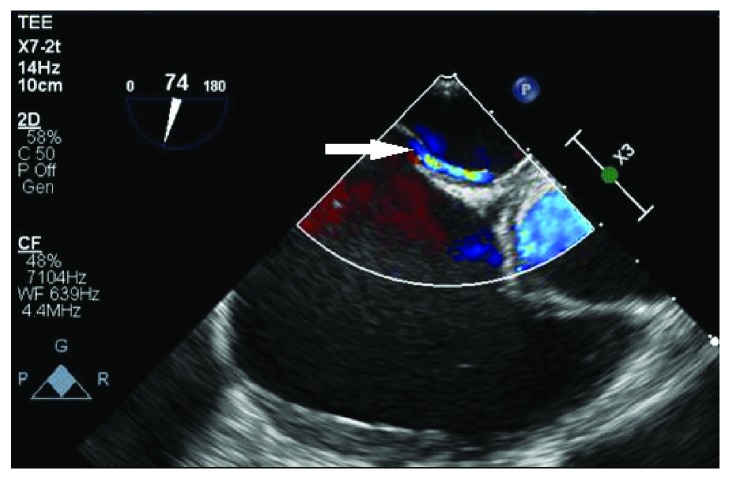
Transesophageal echo showing patent foramen ovale (arrow).
